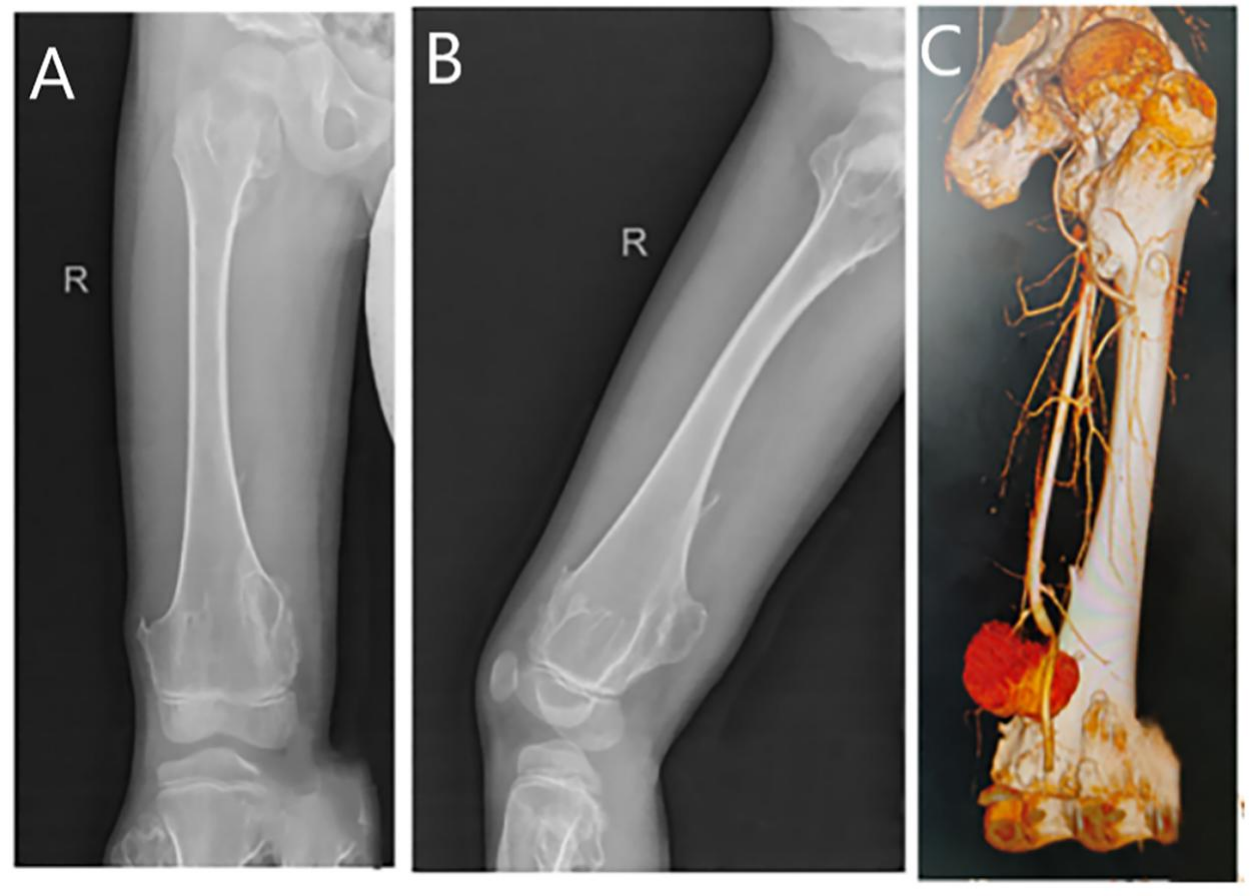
(A) A plain radiograph in the anterior–posterior view reveals an osteochondroma of the right distal femur. (B) A lateral view of the same plain radiograph reveals an osteochondroma of the right distal femur. (C) Computed tomography angiography shows a distal rupture of the right femoral artery with pseudoaneurysm formation.
